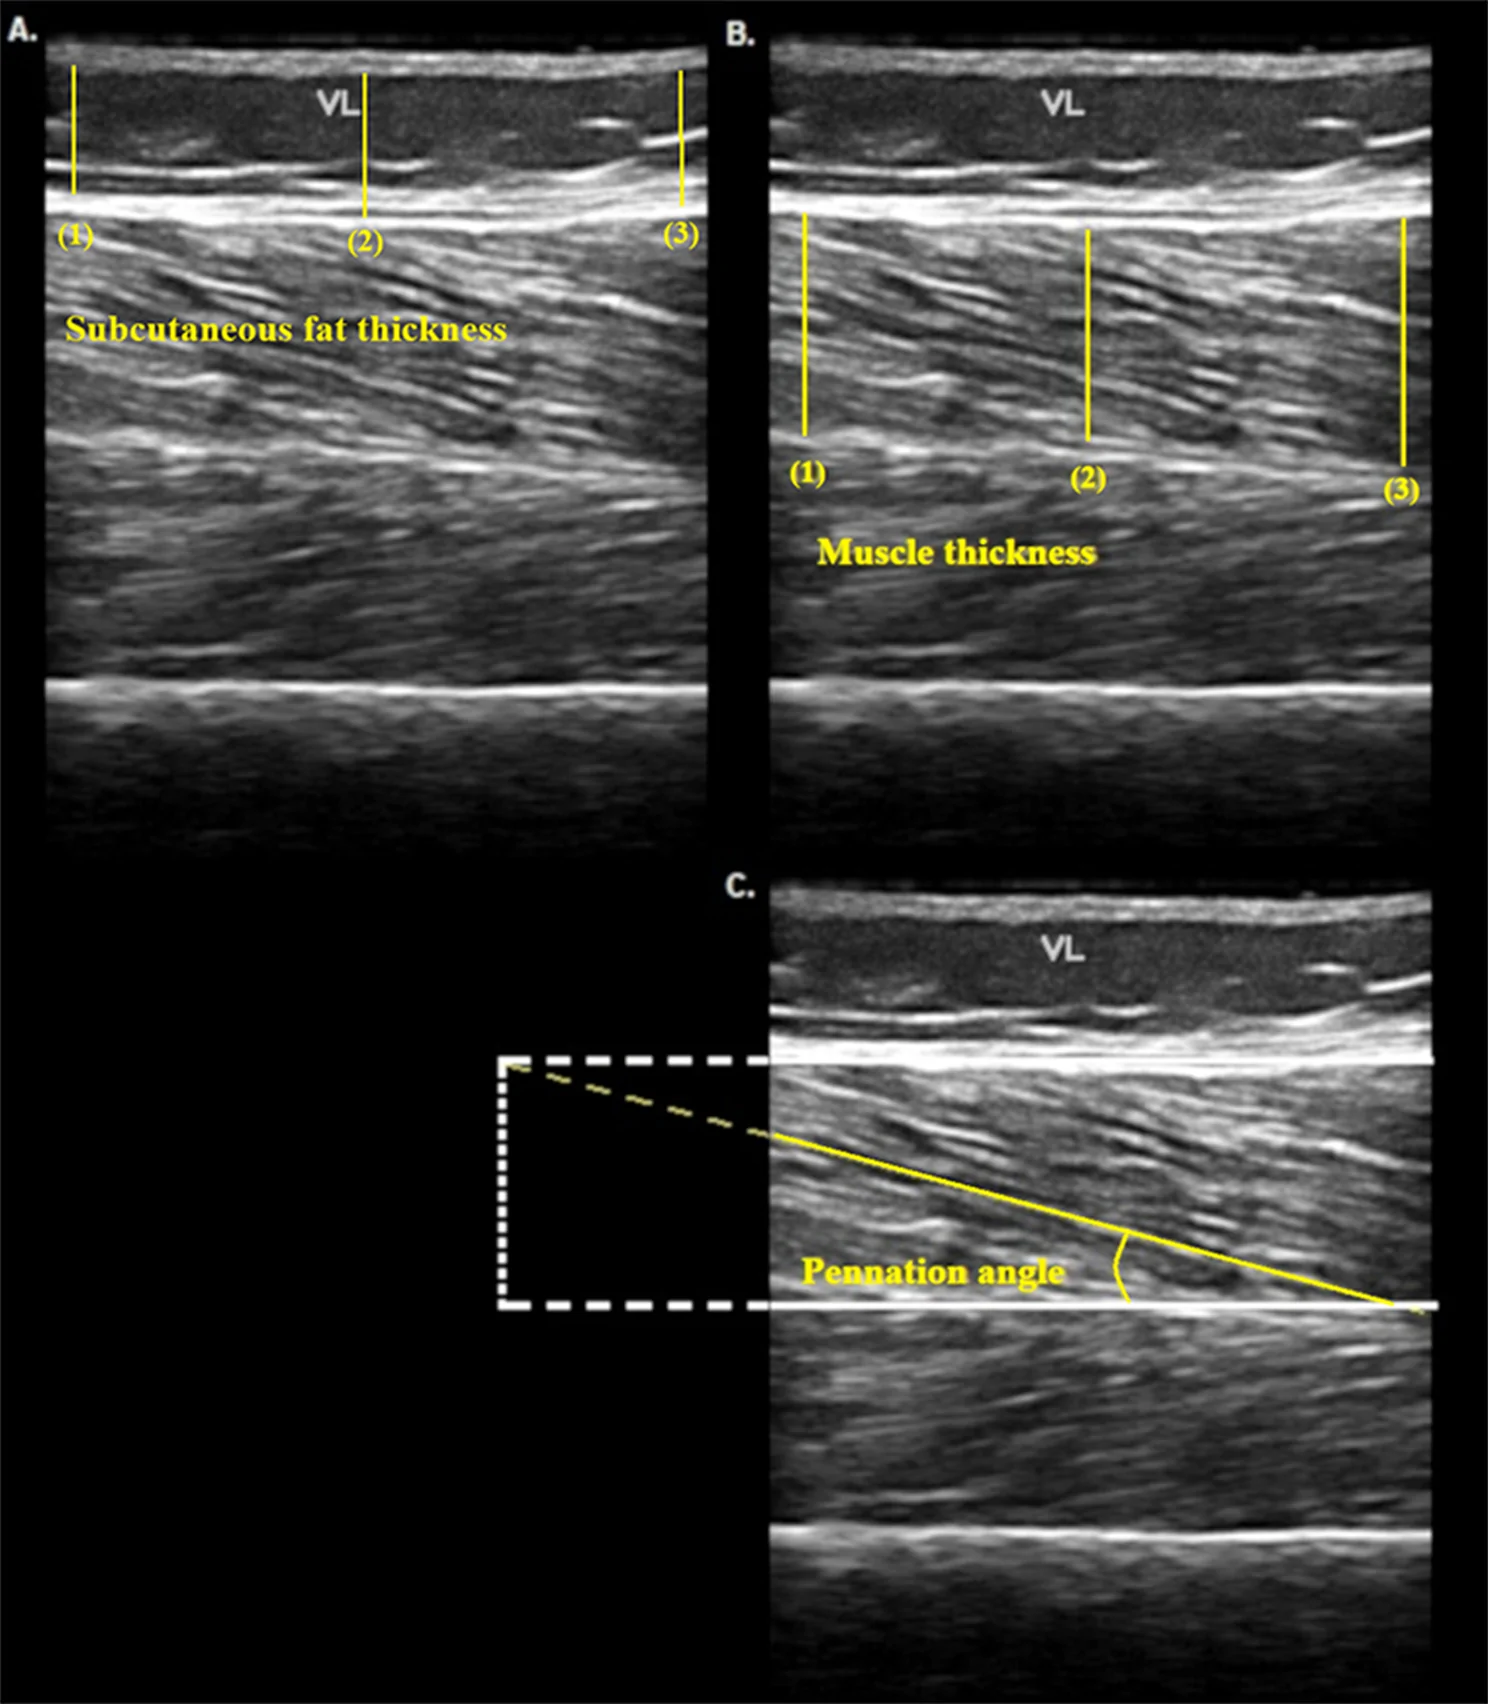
Ultrasound image showing vastus lateralis muscle architecture parameters. (A) assessment of subcutaneous fat thickness, (B) assessment of muscle thickness and (C) assessment of pennation angle. VL: vastus lateralis
